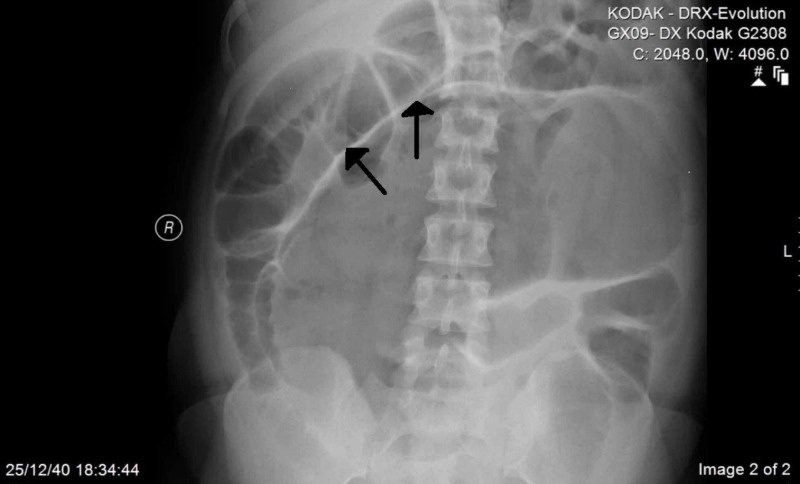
Abdominal radiograph taken in the supine position showing the dilated cecum from the right lower quadrant to the left upper quadrant and the air-fluid level
